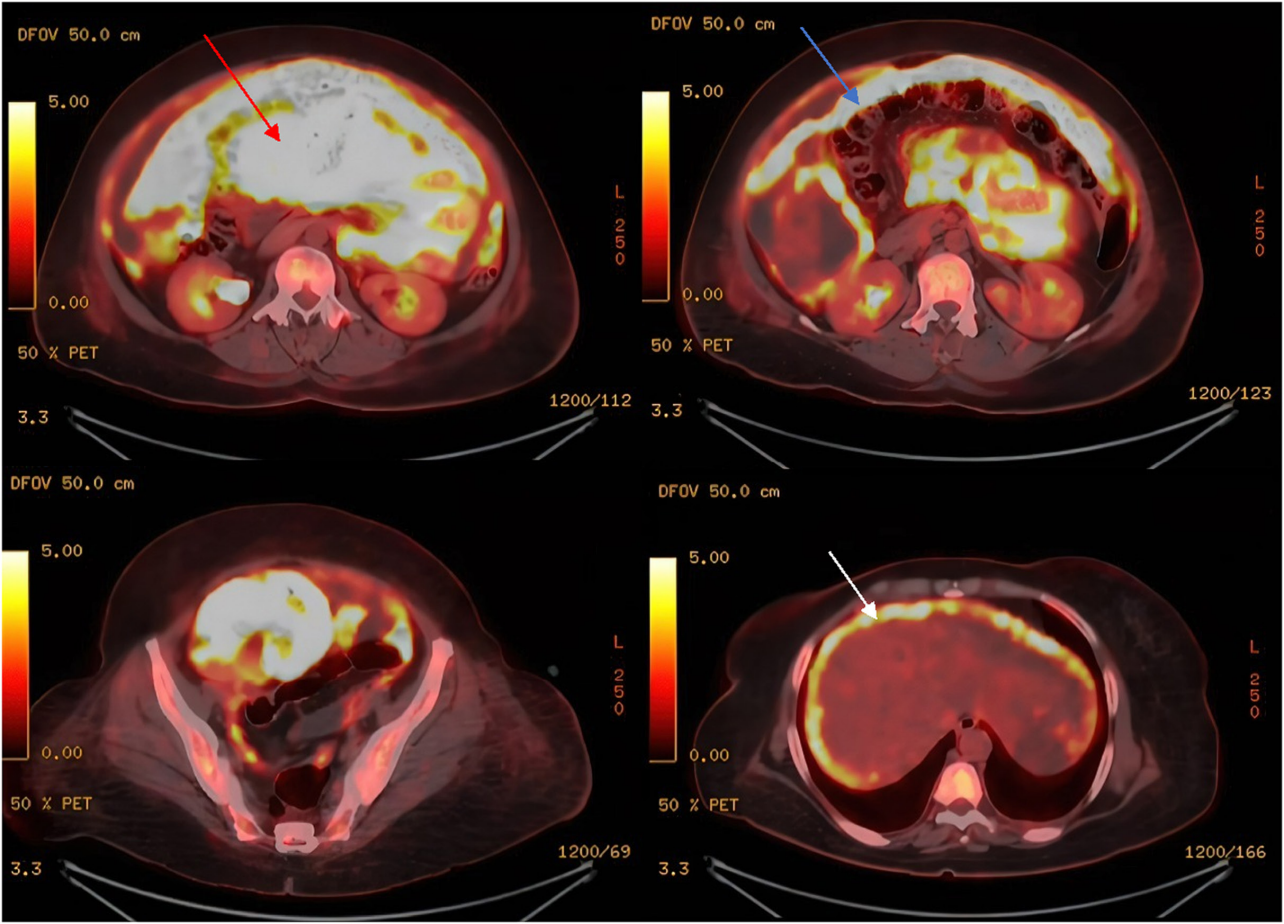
Cross‐sectional (transverse) abdominal/pelvis PET/CT with FDG. It shows an intensely increased FDG uptake around the peritoneal lining (White arrow) with peritoneal thickening (Blue arrow) and omental caking (Red arrow).
